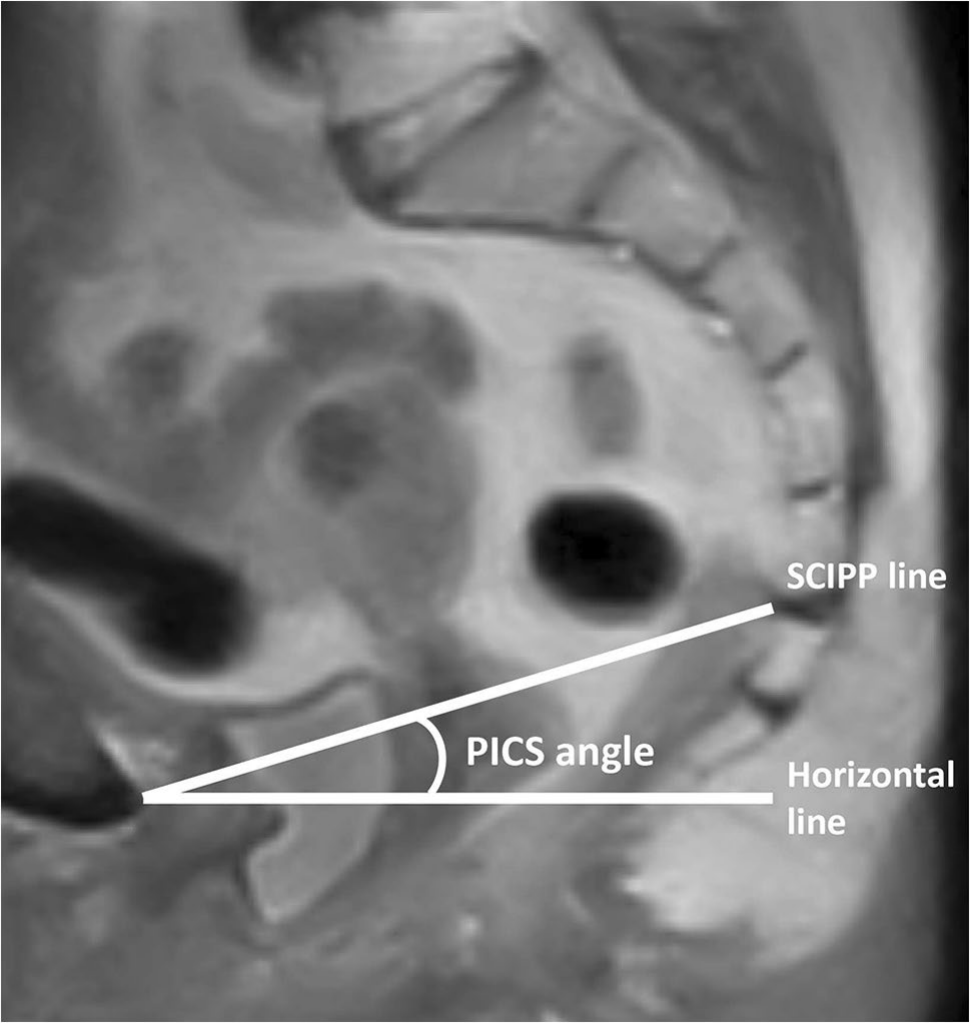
The pelvic inclination correction system (PICS) angle was defined as the angle between the sacrococcygeal–inferior pubic point (SCIPP) line and the horizontal line, based on MRI in the sagittal view. The horizontal line originates at the inferior pubic point and is oriented perpendicular to the longitudinal body axis established by the scanner bed
